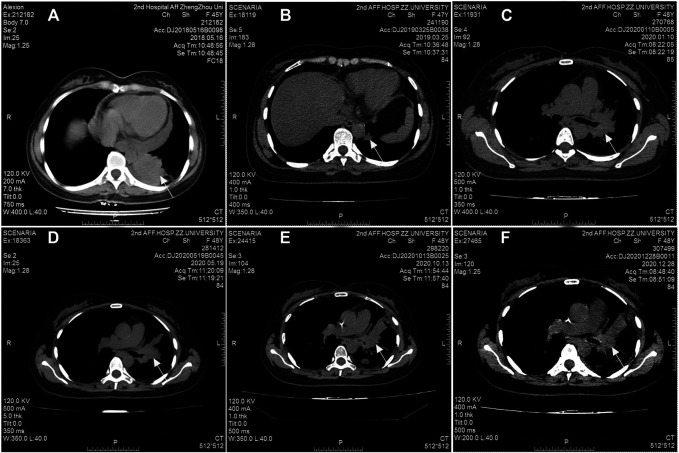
Radiographic response to treatment. Computed tomography (CT) scans at initial diagnosis (A), seven months after taking alectinib for treatment (B), 17 months after taking alectinib for treatment (C), and two months after taking ceritinib for treatment (D), before the second chemotherapy cycle (E), before the 5th chemotherapy cycle (F).
